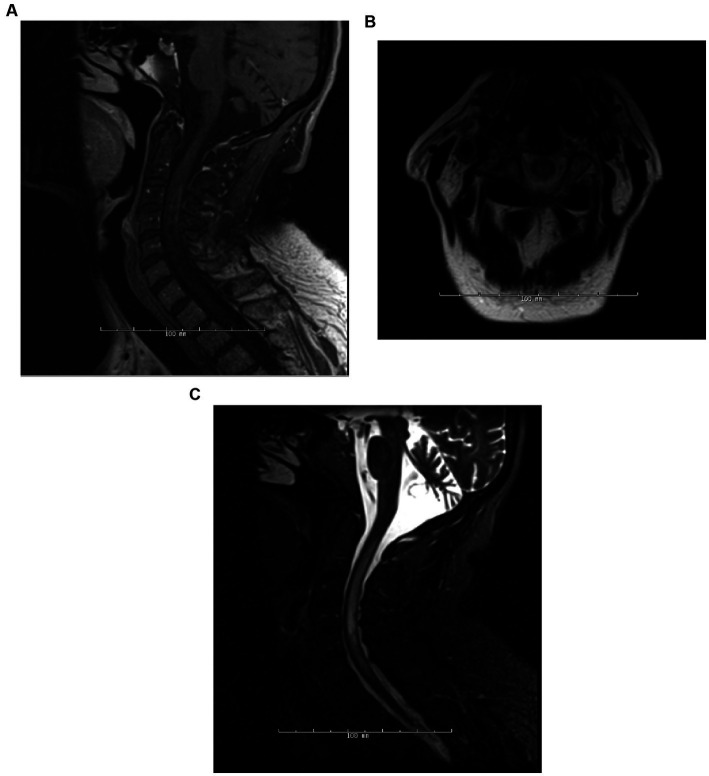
Patient 5 (*detailed under* “*breakthrough disease*” in Supplementary material S3), 6 months post-initiation of rituximab treatment, the patient manifested an attack, encompassing both TM and encephalopathy, with a concurrent CD19+ B-cell count of 3%. MRI assessments during this episode included Sagittal T1-post contrast (A), Axial T2 (B), and Sagittal STIR (C) of the spine.
